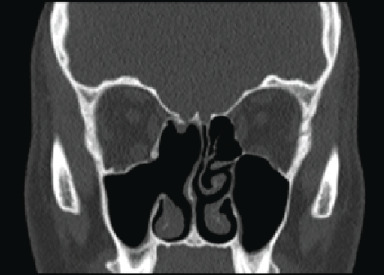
Noncontrast enhanced CT sinuses (coronal section). Postoperative scan demonstrates right ethmoidectomy, middle turbinectomy, and creation of middle meatal antrostomy. Well-aerated sinonasal spaces and no tumour recurrence.
